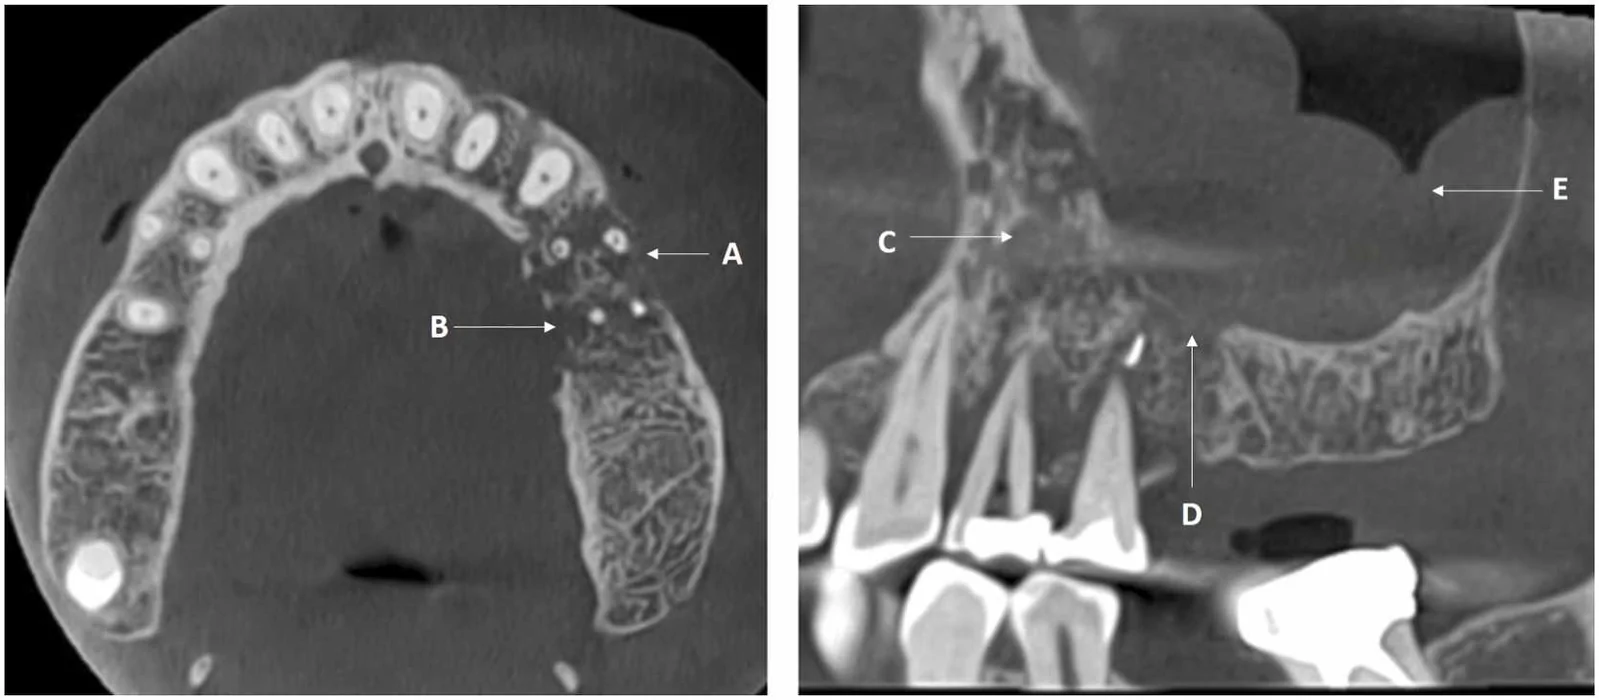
Axial CBCT section showing buccal cortical plate [A] and palatal cortical plate [B] perforation. Sagittal section demonstrating a moth-eaten osteolytic lesion [C] with maxillary sinus floor perforation [D] and thickening of the Schneiderian membrane [E].
